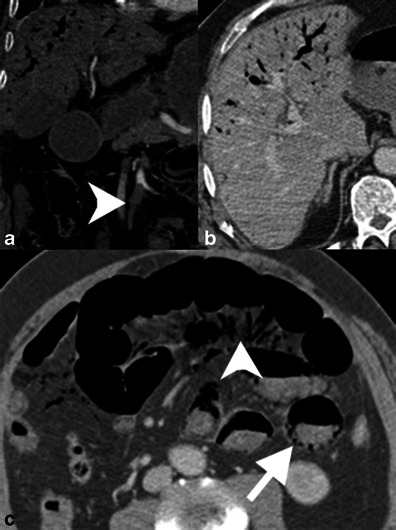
Portomesenteric venous gas. a Contrast-enhanced coronal reformatted CT demonstrates occlusion of the SMA (*arrowhead*). Portal venous gas is seen in the liver. b Axial CT shows portal venous gas in the anti-dependent liver. c The small bowel demonstrates luminal dilation, a paper-thin wall and poorly enhancing mucosa. There is mesenteric venous gas (*arrowhead*) as well as pneumatosis in the dependent bowel wall. The patient had established bowel necrosis and died shortly after imaging
